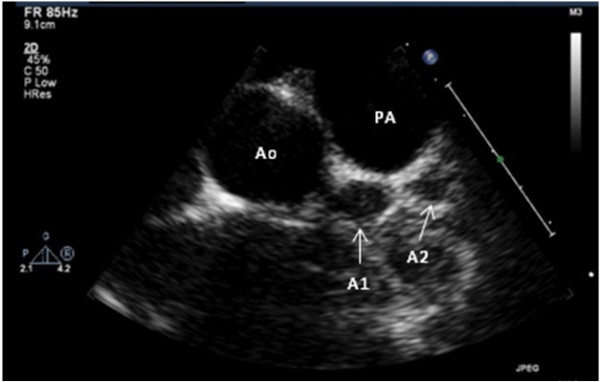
Aneurysms visualized by echocardiography. Echocardiographic parasternal short axis view reveals two fusiform aneurysms of the left anterior descending coronary artery. The proximal aneurysm (A1) measures 8.7 mm, and the distal (A2) measures 9.0 mm. The aorta (Ao) and main pulmonary artery (PA) are seen in cross section.
